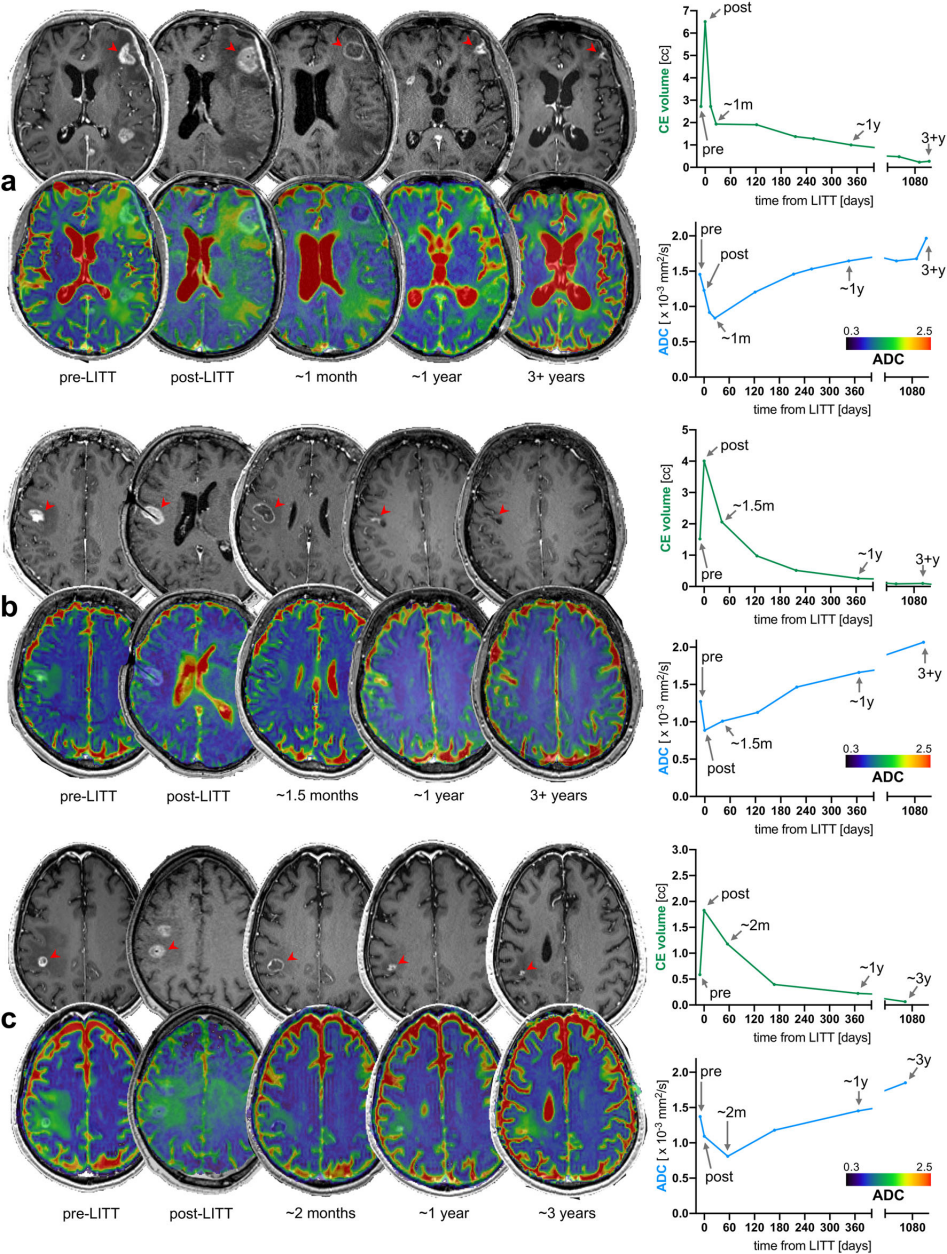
Typical radiographic pattern of durable response. Lesions exhibiting durable response (three representative cases in a–c) typically show an immediate post-LITT enlargement, especially of the CE component, associated with a decrease in ADC values, more pronounced in the central non-CE region. 1–2 months after LITT, the CE component shows a marked volume decrease, while the central non-CE region becomes larger, and ADC values can further decrease or remain stable. In the following months/years, the lesion shrinks more gradually until only some small residual CE tissue remains, while ADC values progressively increase above the pre-LITT values. ADC, apparent diffusion coefficient; CE, contrast-enhancing; pre, pre-LITT scan; post, immediate post-LITT scan
